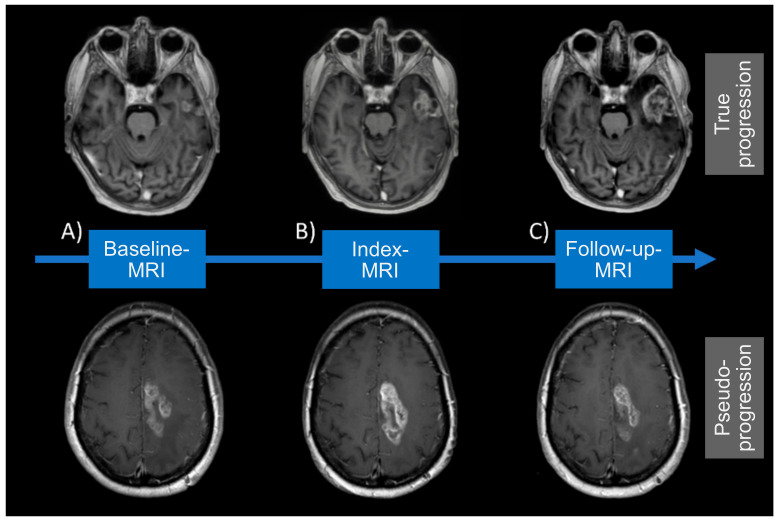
MRI visualization for true progression (TP) and pseudoprogression (PSP). MRI visualization for TP and PSP at (A) Baseline, (B) Index- and (C) Follow-up-MRI. True progression displays an increasing contrast-enhancement at Index- (B) and Follow-up MRI (C). Whereas pseudoprogression shows an enlarging contrast-enhancement at Index-MRI (B) but decreasing lesion on follow-up MRI (C).
